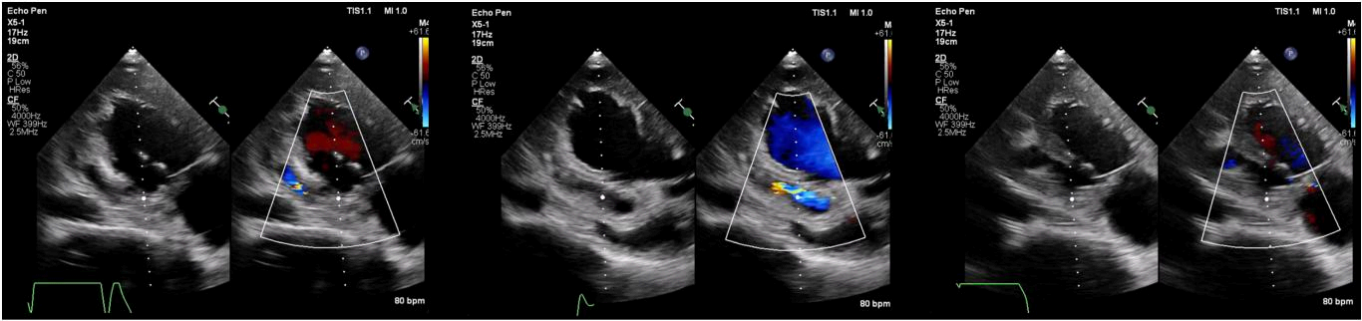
Transthoracic echocardiography, colour Doppler imaging/colour compare view. During the second hospitalization in 2021. Left image: apical two-chamber view, dissection and perfusion of left ventricular wall; middle image: apical three-chamber modified view, dissection and perfusion through left ventricular wall; right image: apical two-chamber view, ostium of dissection and pseudoaneurysm.
